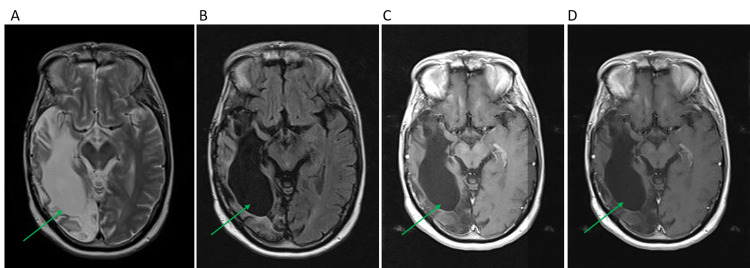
Pre- and Post-Contrast Brain MRI Brain MRI was done for the patient in a follow-up visit after 20 years of the GBM tumor resection. Encephalomalacia is seen in the right frontotemporal lobe. No evidence of glioblastoma recurrence. A: axial flair T1, B: axial T2, C and D: post-contrast axial T1.
